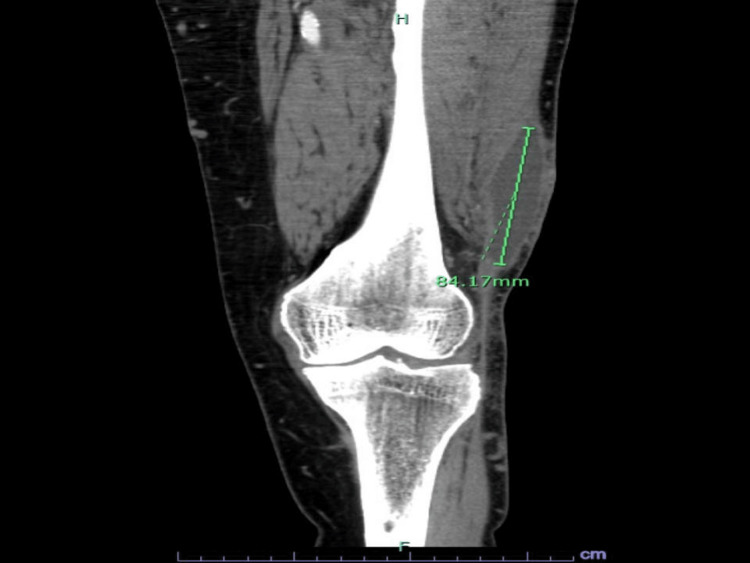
Left knee CT with contrast showed a postoperative seroma along the distal and lateral aspect of the left thigh superficial to the vastus lateralis musculature.
